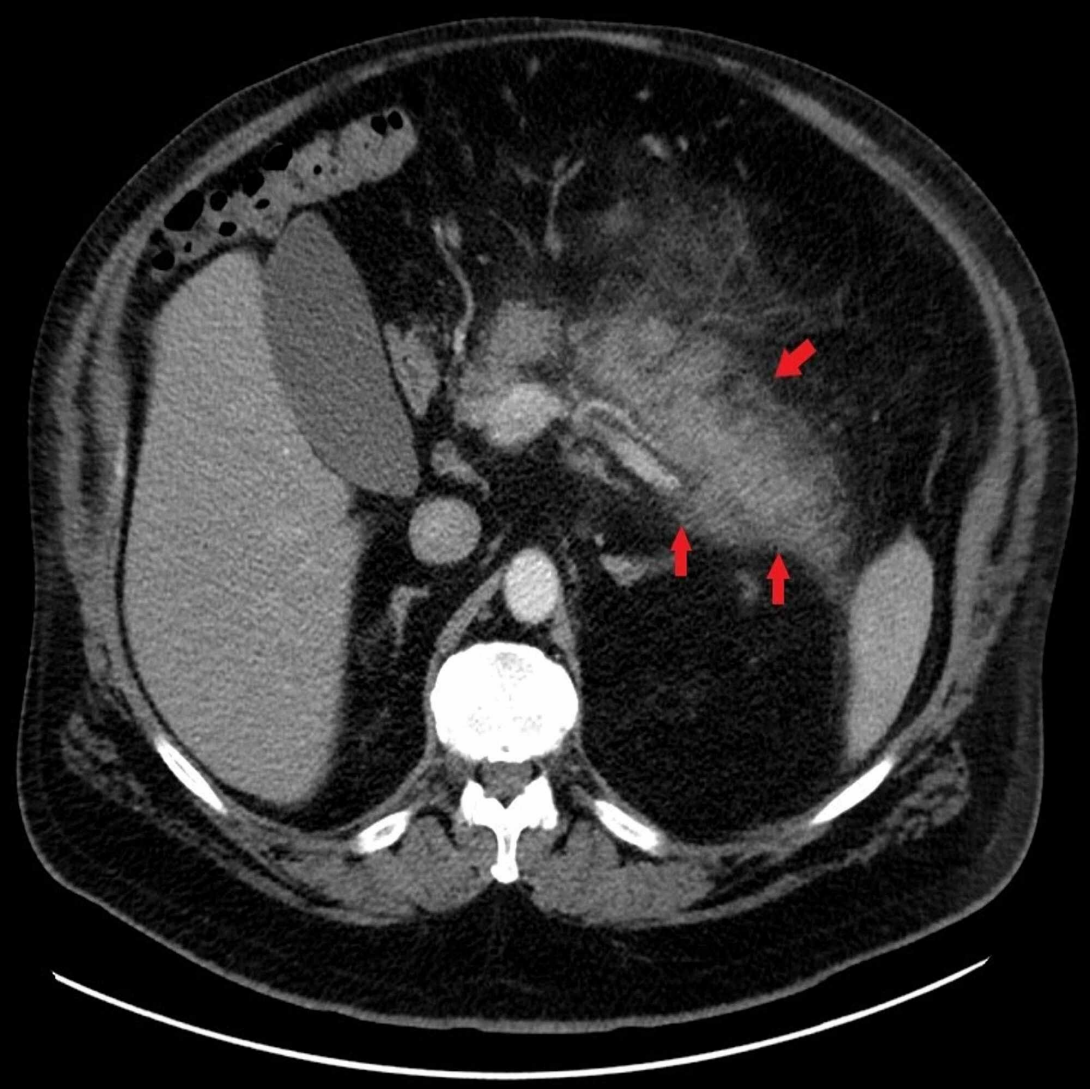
CT abdomen without contrast demonstrating diffuse peripancreatic stranding
